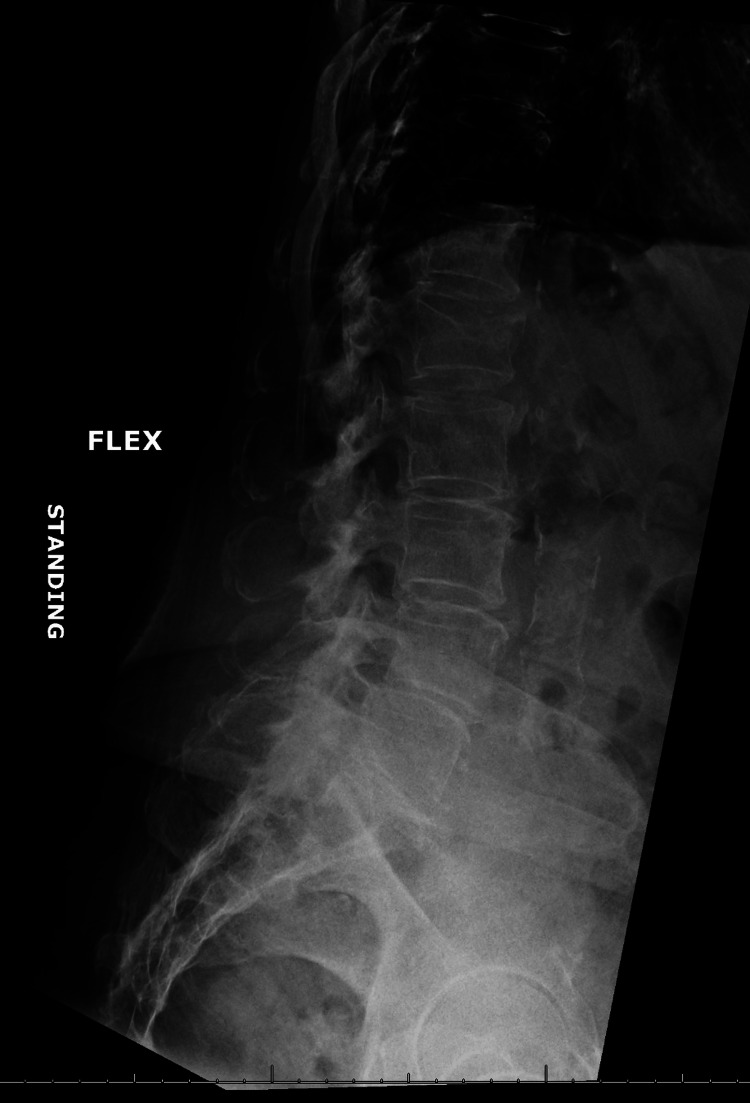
Lumbosacral X-ray flexion view showing improvement of retrolisthesis of L1 on L2 and L2 on L3.
